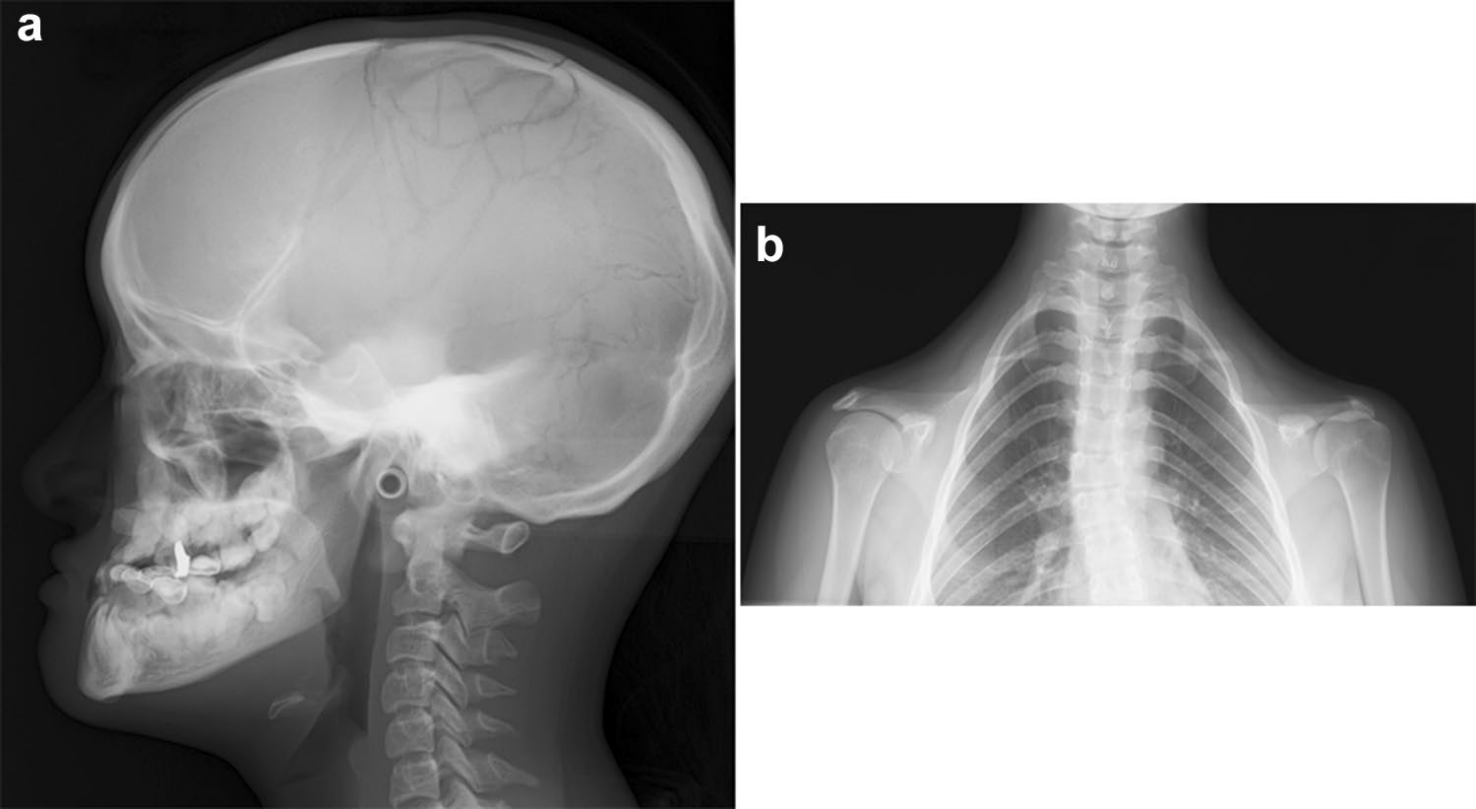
a, b A 25-year-old female with cleidocranial dysplasia. Radiographs show multiple Wormian bones, absent bilateral clavicles, and a narrow upper thorax
